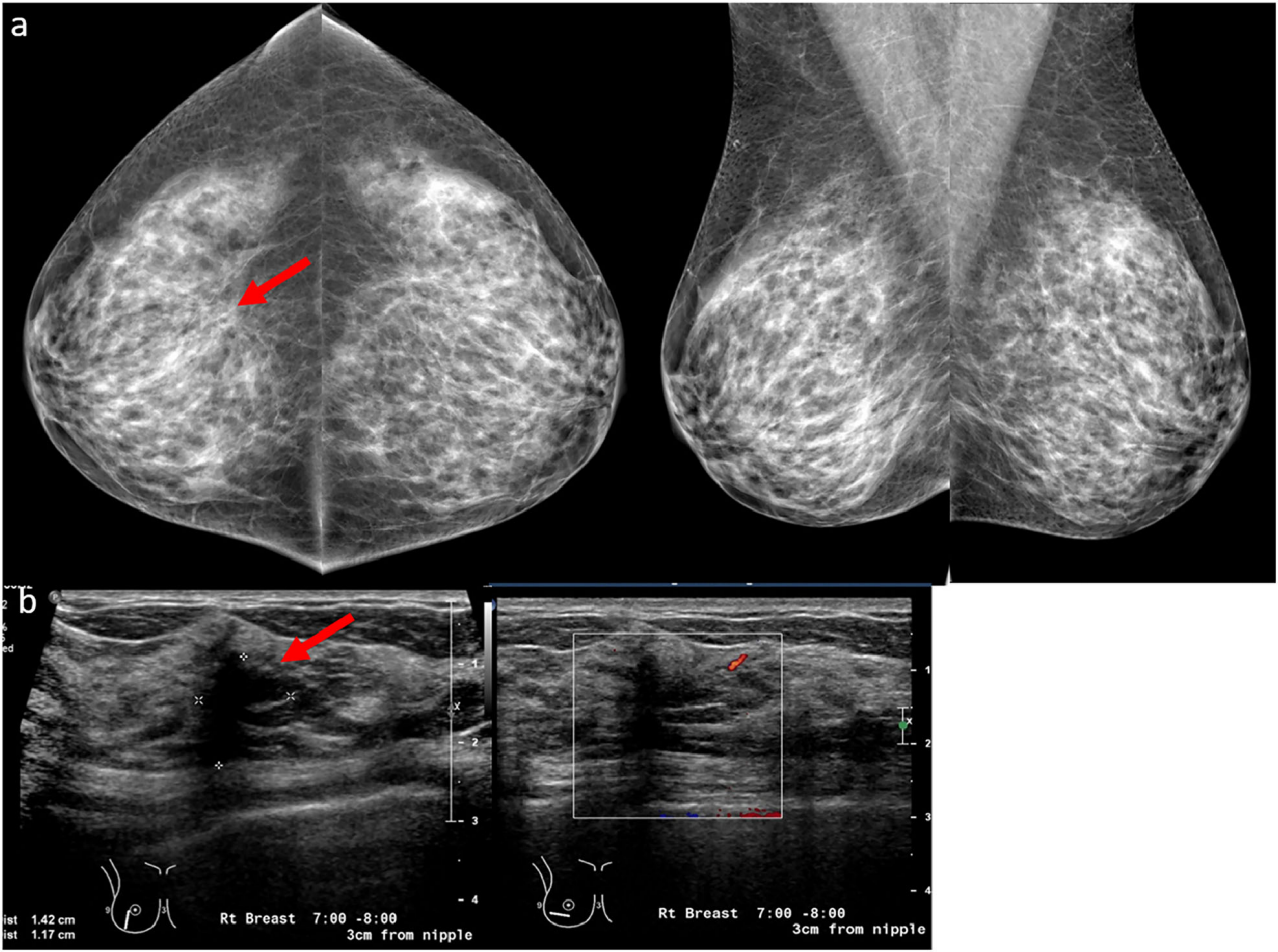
a CC and MLO screening mammograms in a 41-year-old woman revealed a subtle architectural distortion in the central right breast seen on the CC view only (red arrow). This finding was not reported by one reader. However, the second reader identified the abnormality and recommended a recall for further assessment. At our institution, all screening mammograms are routinely interpreted by two independent readers. b Targeted ultrasound revealed a focal hypoechoic non-mass lesion (red arrow) in the corresponding right breast, with associated posterior shadowing and internal vascularity. Ultrasound-guided biopsy revealed grade 1 invasive ductal carcinoma
